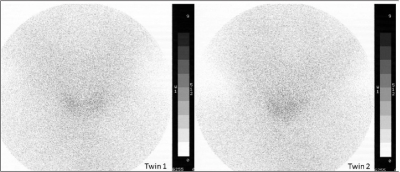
Thyroid hypoplasia without ectopic tissue was detected by technetium 99m pertechnetate thyroid scintigraphy in both twins
